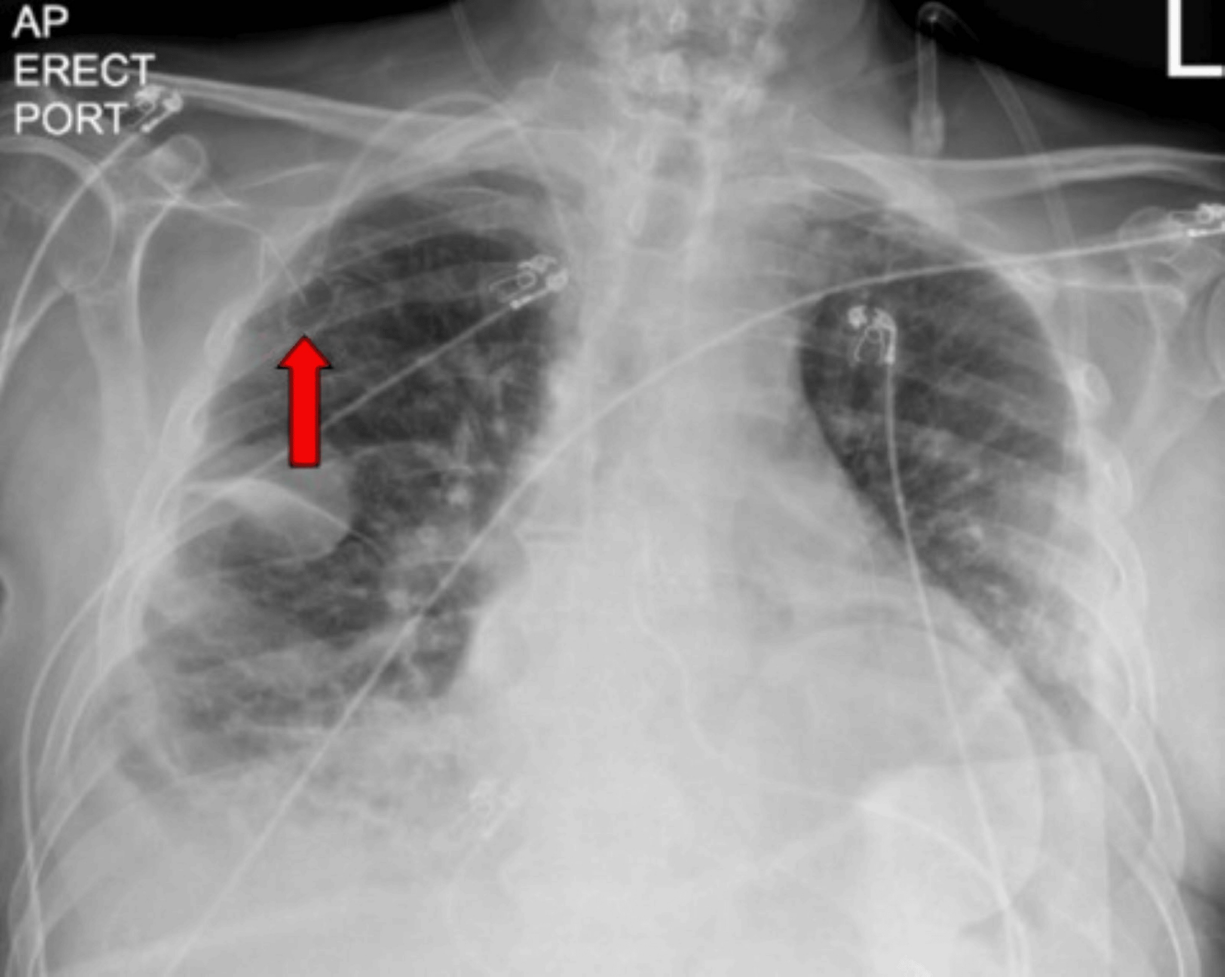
Chest X-ray showed pulmonary edema, a right internal jugular vein chest port (red arrow), and a right lower lobe infiltrate
